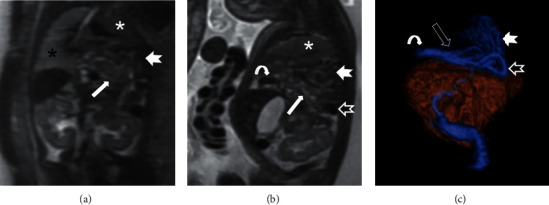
Left diaphragmatic hernia. Gestational age: 27 weeks and 6 days. (a) Coronal SSFSE T2. (b) Sagittal SSFSE T2. (c) Volume rendering coronal reconstruction. Complete herniation of jejunal (solid white arrow) and ileal (solid white arrowhead) loops is observed. Herniation of the hepatic angle of the colon (curved white arrow), ascending colon and cecum (empty white arrow) is also observed, and they are located above the splenic angle of the colon (empty white arrowhead). The left lung is hypoplastic and is collapsed in the left upper hemithorax (white asterisk). The cardiomediastenic displacement towards the right hemithorax and normal right lung (black asterisk) is shown. No hepatic or gastric herniation is observed. Relative pulmonary volume is 72.64%; the intensity ratio of the pulmonary parenchyma relative to the hepatic parenchyma is >1 and the intensity ratio of the pulmonary parenchyma in relation to the CSF is 0.78, implying a good prognosis. Fetal MRI provided useful information for perinatal management and prognosis over the fetal ultrasound.
